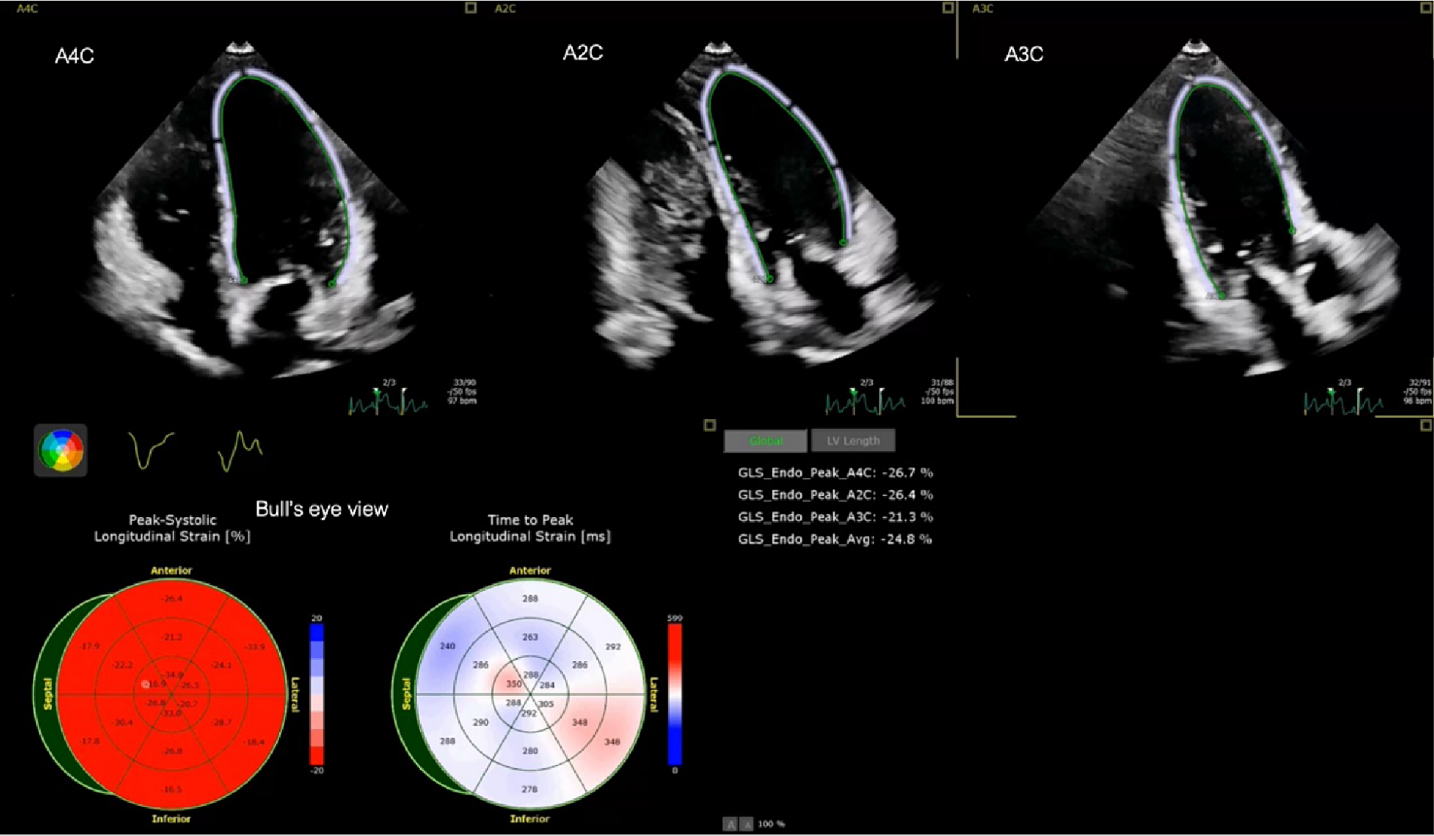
Example of left ventricular strain of a child with DRE including apical four chamber (A4C), apical two chamber (A2C), apical three chamber (A3C) and average strain (Avg) strain in Bull’s eye view.
